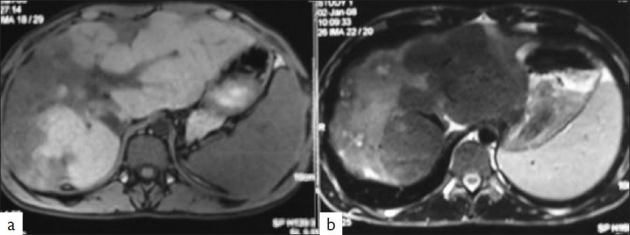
MRI images (T1-weighted) showing a diffuse poorly defined hypointense mass in right and left lobes of liver with subcapsular retraction (a). The mass is hyperintense on T2-weighted image (b)
